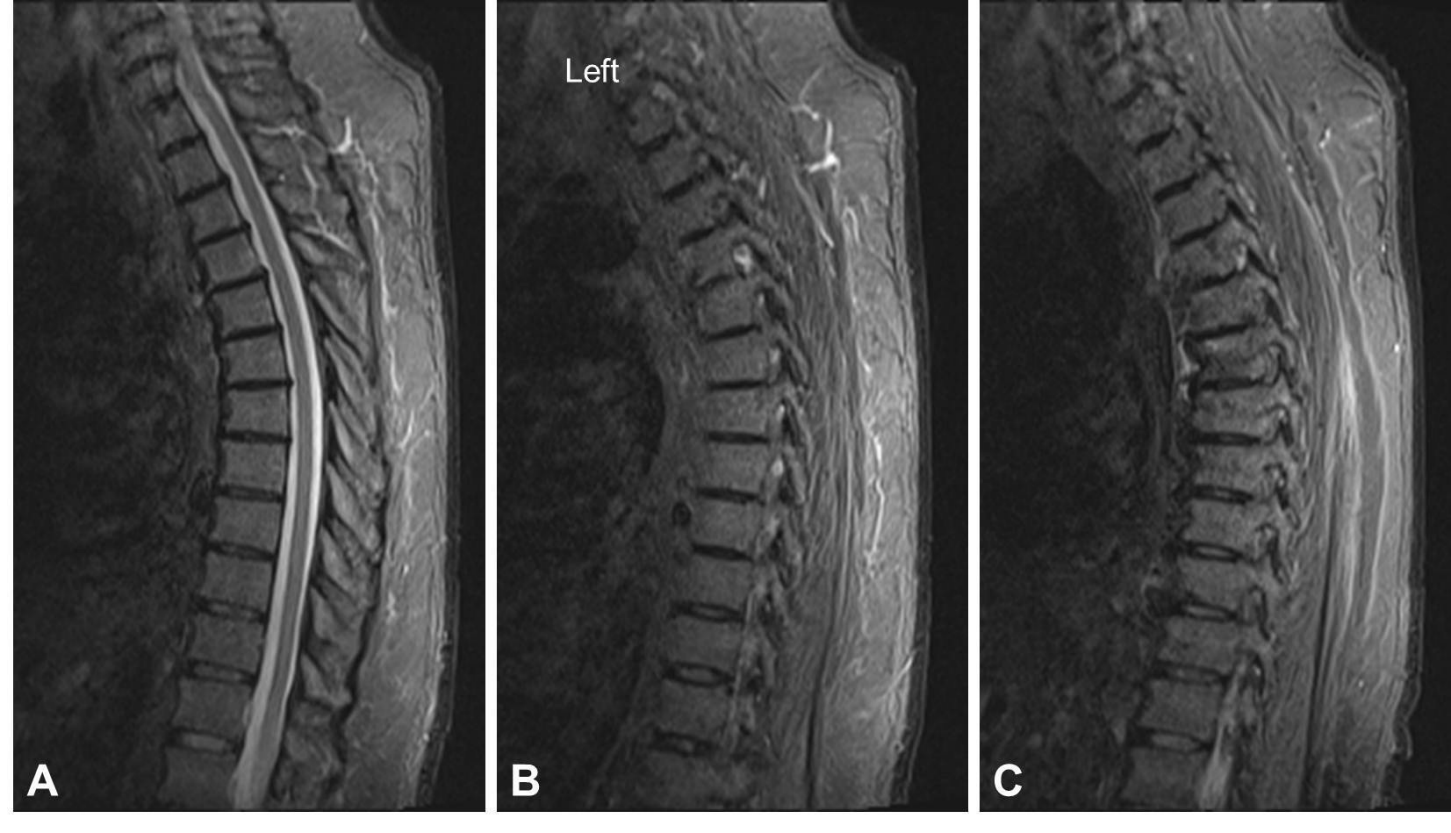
MRI Following Recumbency Treatment An MRI short tau inversion and recovery (STIR) sequence demonstrates normal signal intensity at the level of the anterior longitudinal ligament, disk, and posterior ligamentous complex (interspinous and supraspinous ligaments) at the level of T4/5 and bone osteophyte formation (A). A left (B), and right (C) parasagittal image shows normal signal intensity at the level of previously disrupted facet joints.
